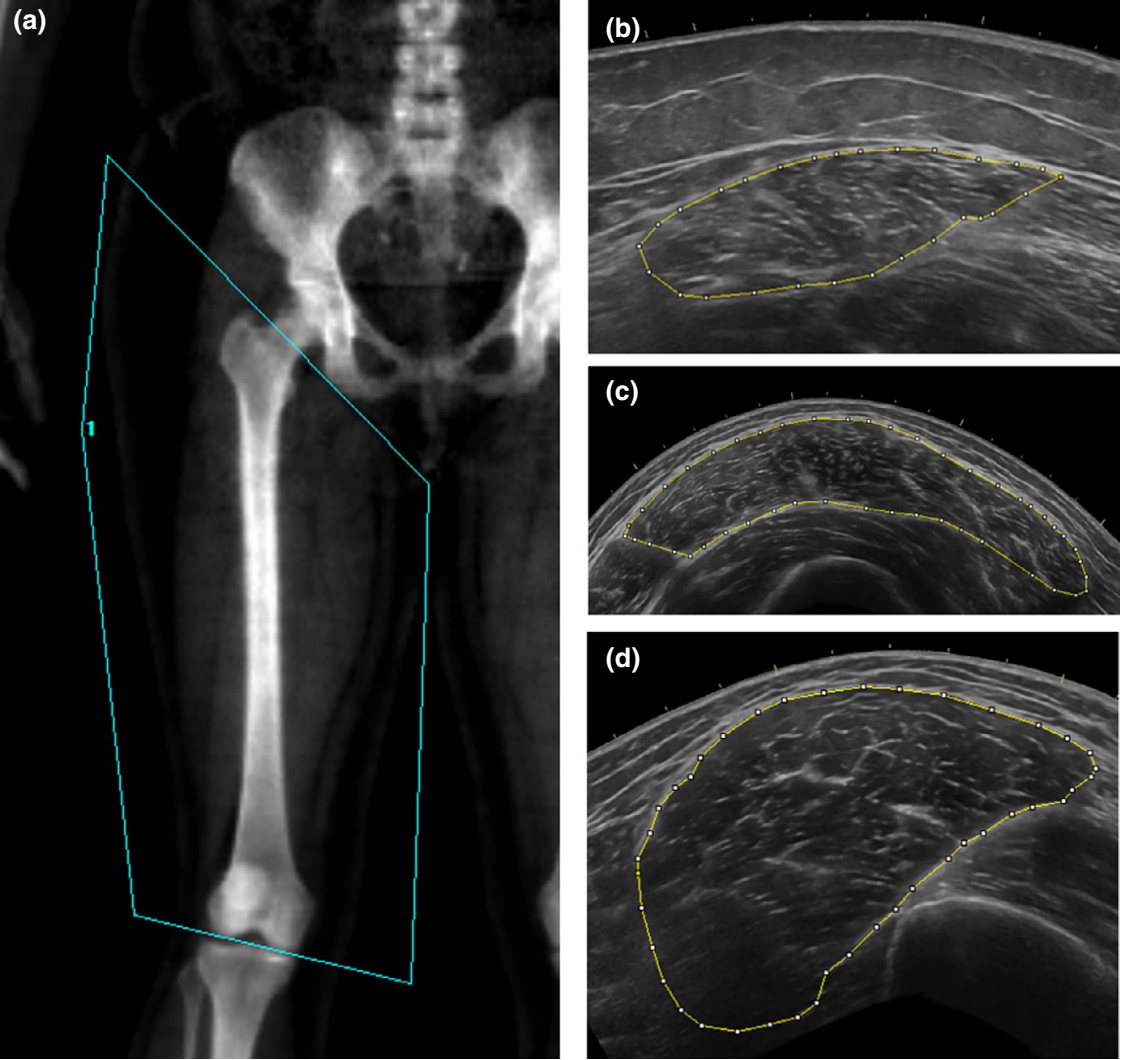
(a) Thigh lean mass region‐of‐interest. Ultrasound cross‐sectional area of the (b) rectus femoris, (c) vastus lateralis, and (d) vastus medialis
